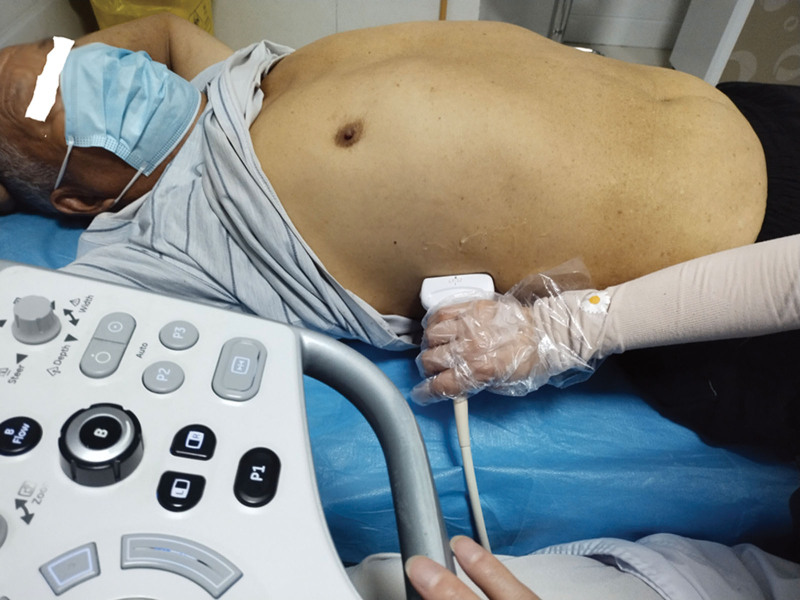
Examination position of the patient and position of the ultrasound probe.
